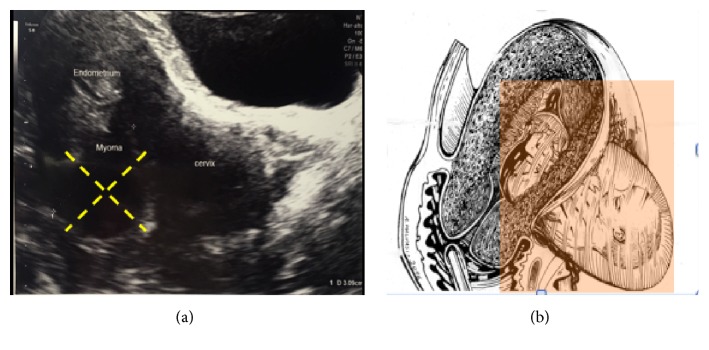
(a) 2D transvaginal ultrasound of the IM isthmic myoma type 5. (b) Graphic depiction of the herniated fetus through the isthmic defect on the previous myomectomy scar.
